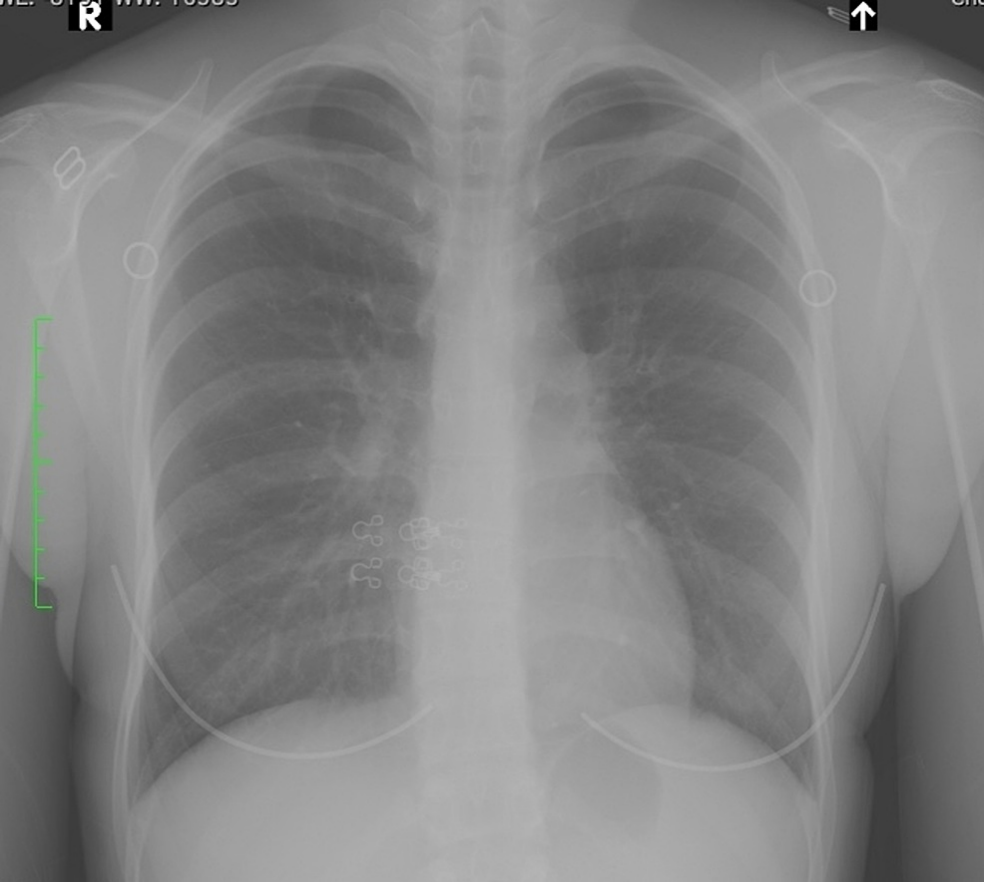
Chest X-ray Chest X-ray showed no abnormal findings such as obvious infiltration shadows or consolidative change. R: right side.
